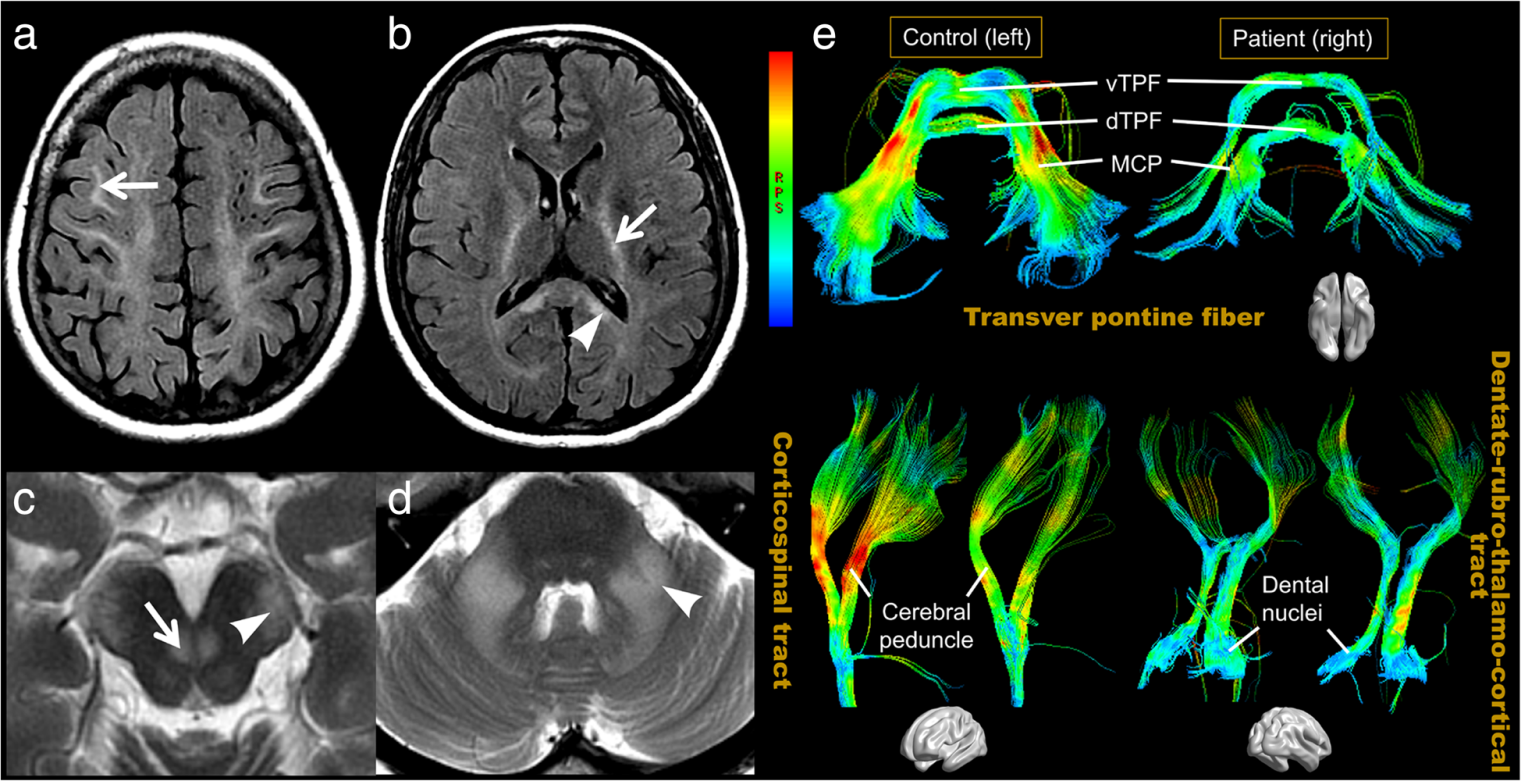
Conventional brain magnetic resonance imaging (MRI) and diffusion tensor tractographies (DTTs) of the patient. Axial T2-FLAIR (a, b), and T2-weighted (c, d) MRI of the patient demonstrate abnormal hyperintensities in the bilateral frontal (a, arrow) and parietal white matter and the splenium (arrowhead, b) of the corpus callosum, internal capsule (arrow, b), cerebral peduncle (arrowhead, c), DSCP (arrow, c), and middle cerebellar peduncle (arrowhead, d). On DTT (e), the CST, transverse pontine fibers, and dentate nucleus tracts of the patient are obviously thinner and have a “darker color” in the pathological tracts, which indicates lower fractional anisotropy (FA) values, when compared with those in a control. *DSCP = decussation of the superior cerebellar peduncle, CST = corticospinal tract, vTPF = ventral transverse pontine fiber, dTPF = dorsal transverse pontine fiber, MCP = middle cerebellar peduncle*
